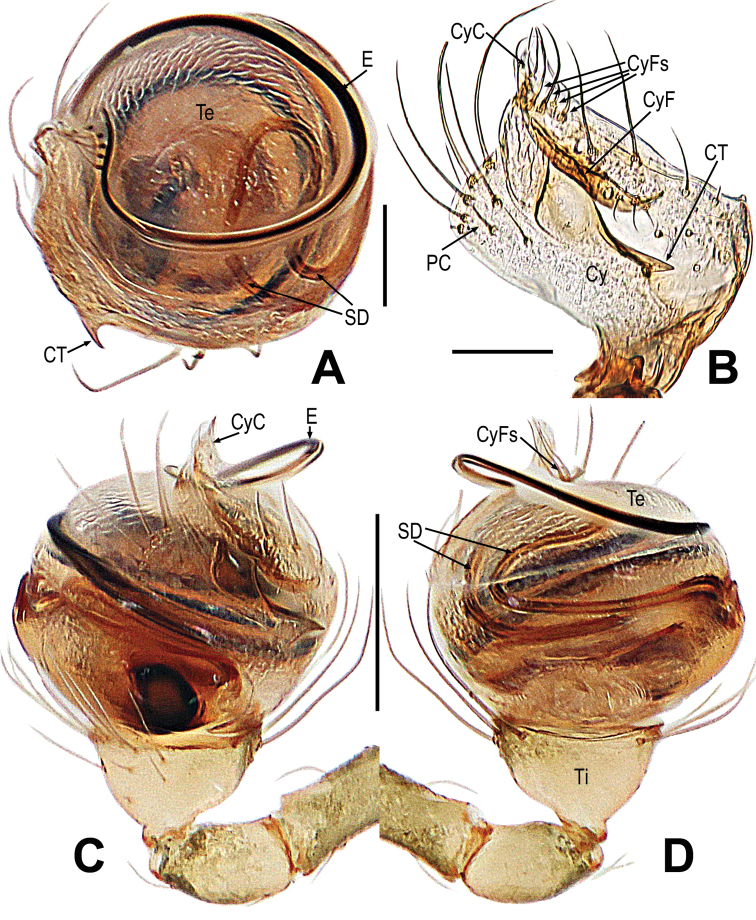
Mosuzhengi* comb. nov. A, C, D male palp B cymbium. A apical B proventral C prolateral D retrolateral. Abbreviations: Abbreviations: CT = cymbial tooth; Cy = cymbium; CyC = cymbial conductor; CyF = cymbial fold; CyFs = setae on cymbial fold; E = embolus; PC = paracymbium; SD = spermatic duct; Te = tegulum; Ti = palpal tibia. Scale bars: 0.10 mm (A, B); 0.20 mm (C, D).
